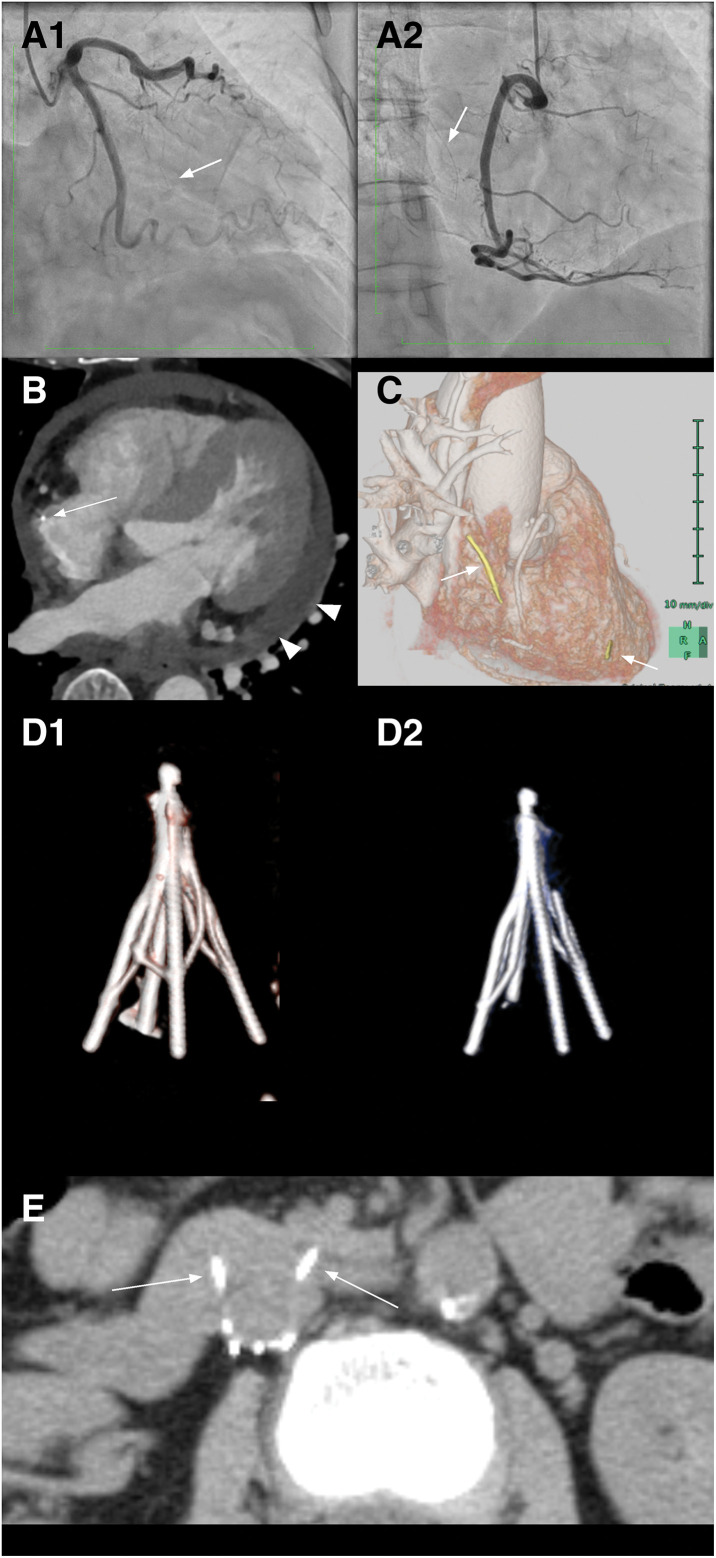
Preoperative imaging findings. (A) CAG showing a linear radiopaque structure near the cardiac silhouette (arrows). (A1) Left CAG. (A2) Right CAG. (B) Contrast-enhanced CT showing pericardial effusion (arrowheads) and a hyperdense structure within the RA (arrow). (C) 3D CT reconstruction demonstrating hyperdense fragments located in the RA and around the RV, showing a strut-like morphology. (D) 3D CT comparison of the IVC filter. (D1) 3D image obtained 4 years earlier demonstrating an intact IVC filter configuration. (D2) Current 3D image demonstrating a defect in the filter structure, consistent with filter fracture. (E) Contrast-enhanced CT showing penetration of the IVC filter through the IVC wall into the duodenum (arrow). CAG, coronary angiography; IVC, inferior vena cava; RA, right atrium; RV, right ventricle
